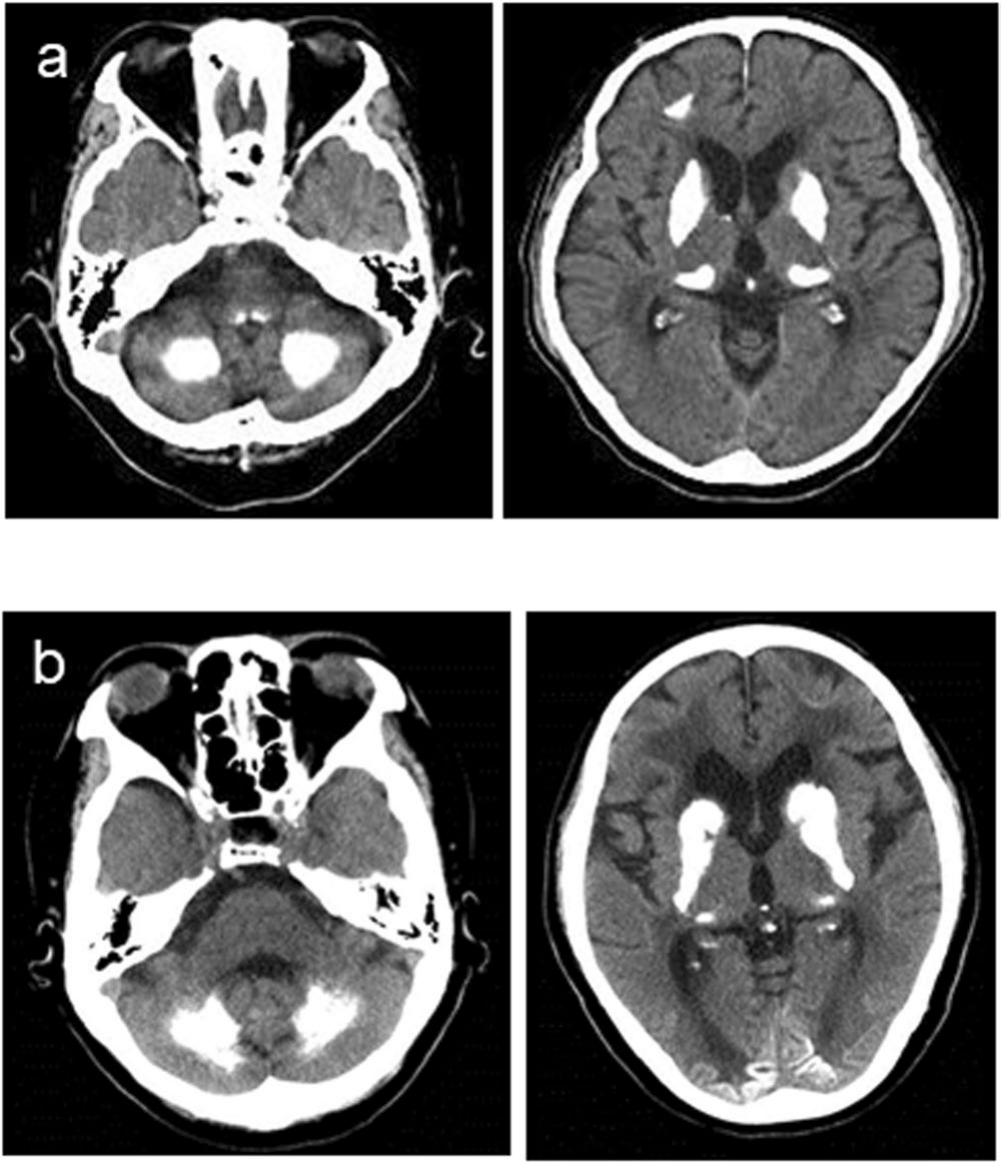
CT images. (a) Computed tomography (CT) images of Case 3 with PDGFB deletion variant (c.33_34delCT). (b) CT images of Case 4 with PDGFB insertion variant (c.342_343insG).
